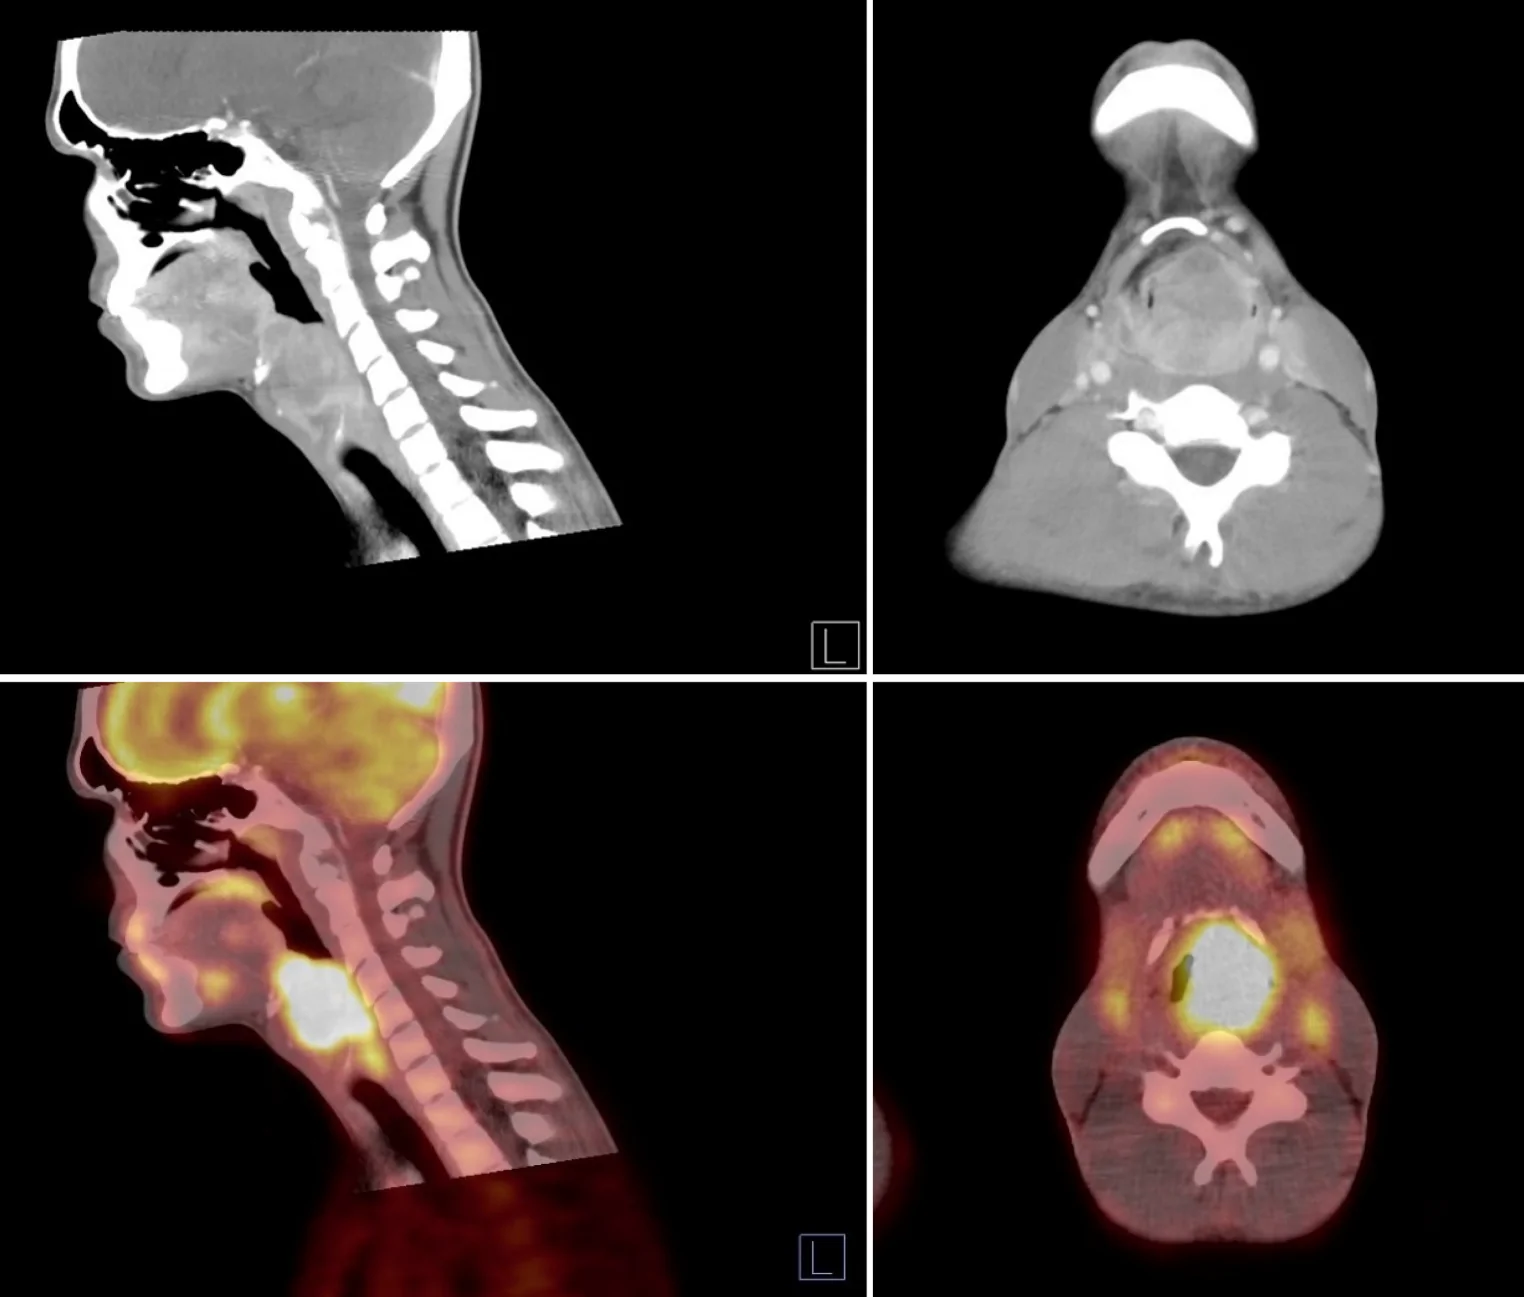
Baseline imaging. Contrast-enhanced computed tomography (CT) demonstrating a left supraglottic mass crossing the midline with extension to the hypopharynx and tongue base. ^18^F-fluorodeoxyglucose (FDG) positron emission tomography/CT (PET/CT) (fused) showing an FDG-avid supraglottic primary with bilateral cervical nodal uptake
